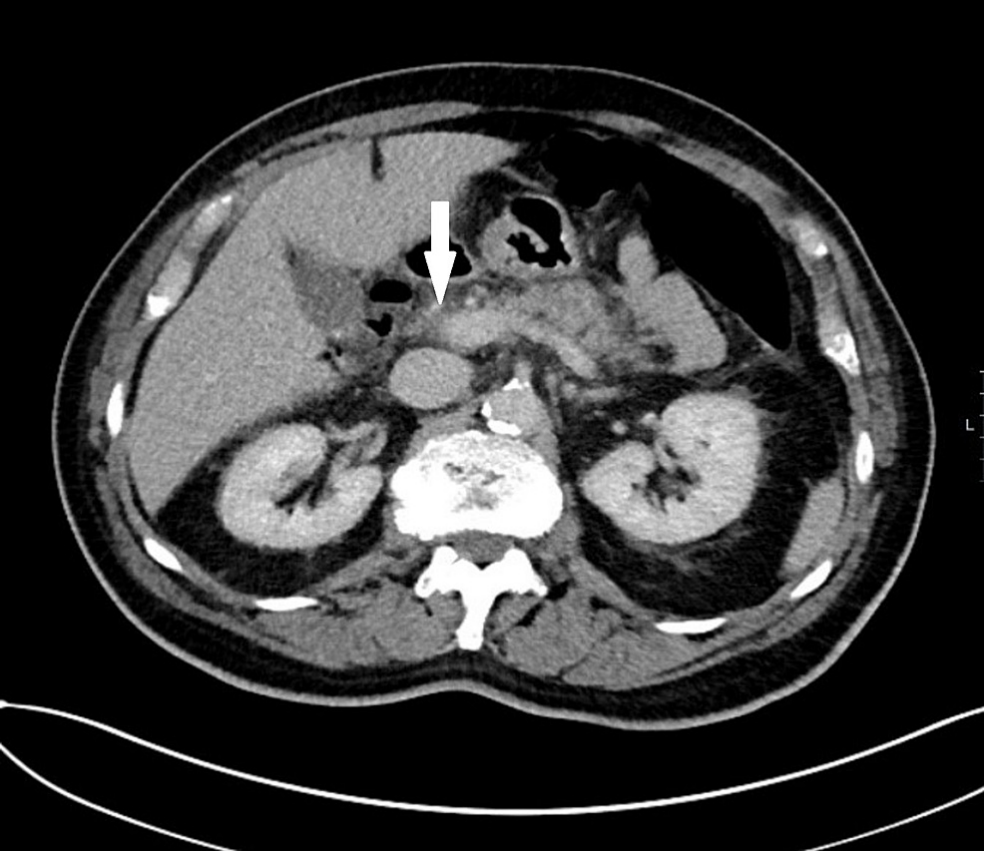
CT scan showing mild pancreatitis (see white arrow).
